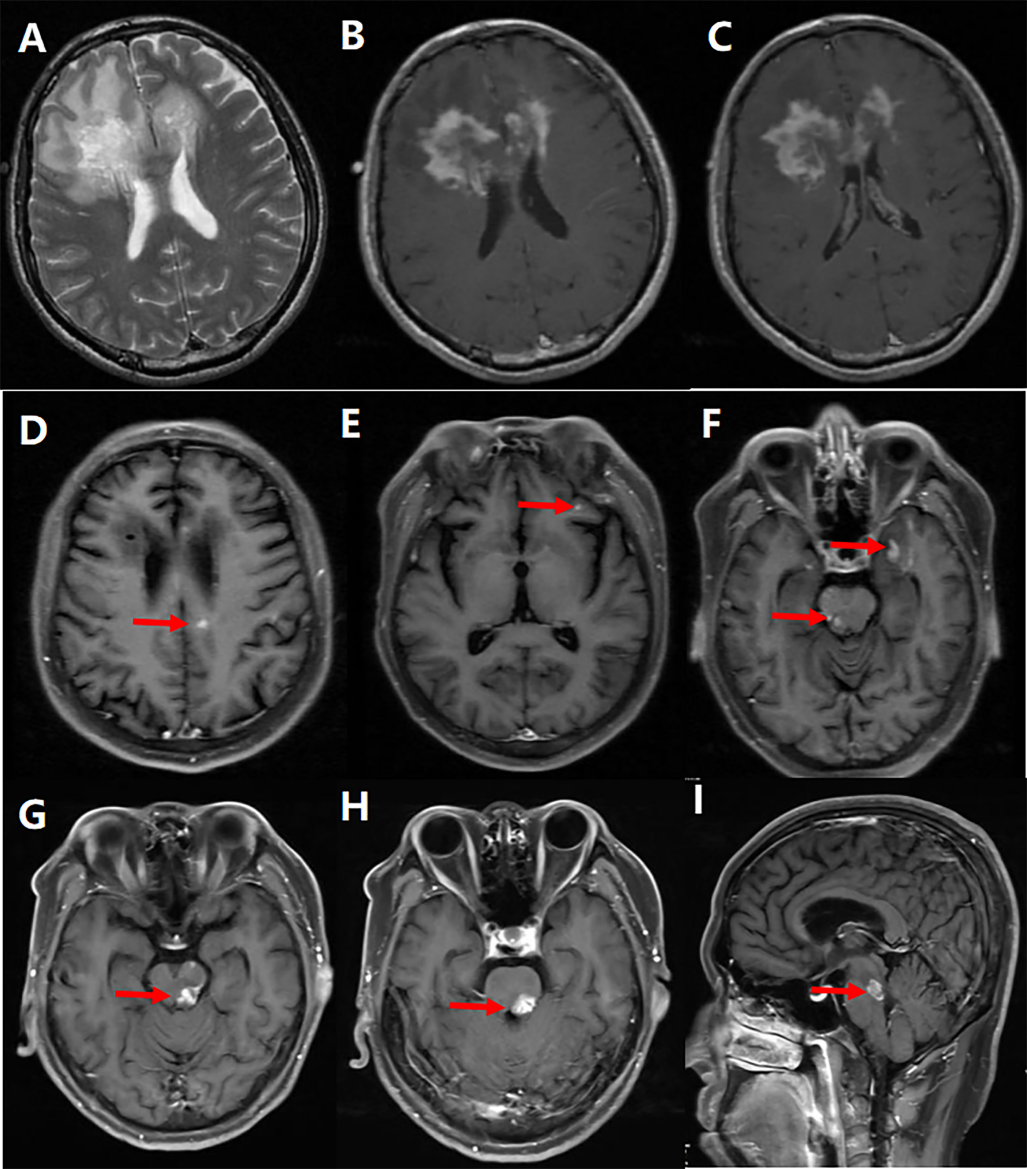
Representative example of tumefactive multiple sclerosis (MS). A 44-year-old man’s MRI scan at the first attack revealed an extensive hyperintensity on T_2_-weighted (A) and contrast-enhanced T_1_-weighted (B, C) images extending from the left frontal lobe through the corpus callosum knee to the left frontal lobe, and the lesions on the contrast enhancement images gradually disappeared after treatment; 7 months after the onset, some small patchy lesions developed in the right lateral paraventricular (D), frontal (E), temporal, and left midbrain (F), which gradually disappeared after treatment; The second relapse occurred 19 months after the first episode, and the patient’s brainstem developed a new patchy lesions (G–I).
